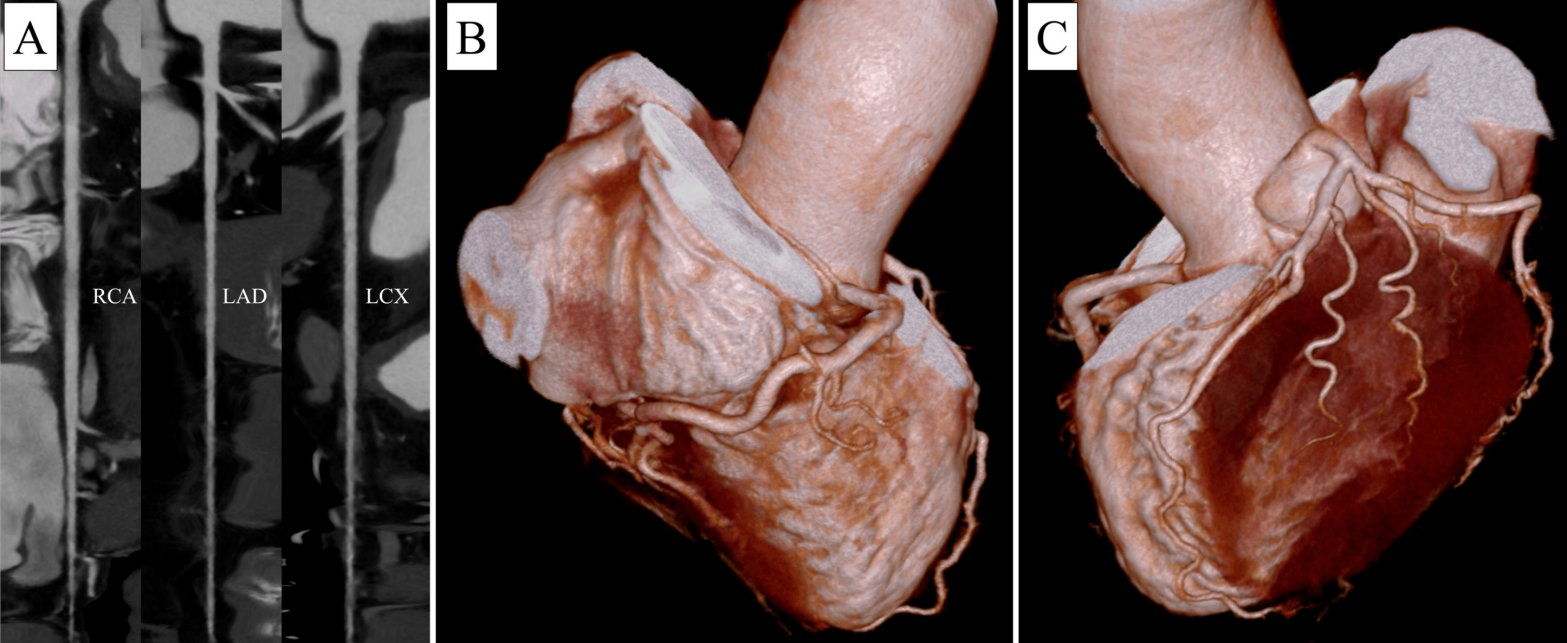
CT angiography image. CT angiography image showing no stenosis in the coronary arteries. (A) CT angiography showing the three main coronary arteries reconstructed from contrast-enhanced CT images. (B) Three-dimensional reconstructed image of the right coronary artery region. (C) Three-dimensional reconstructed image of the left coronary artery region.
